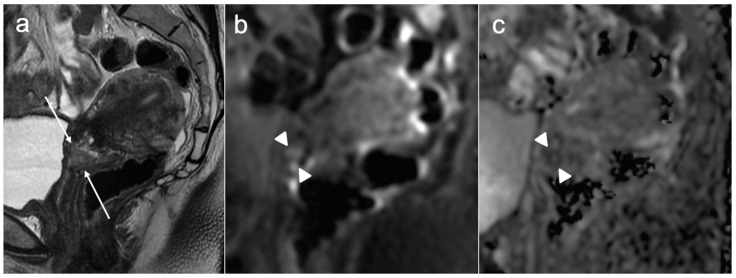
Sagittal T2WI (a) demonstrates a 15 mm intermediate signal intensity lesion in the anterior lip of the cervix (arrows). Corresponding DWI (b) and ADC map (c) show associated restricted diffusion (arrowheads). FIGO stage IB1.
